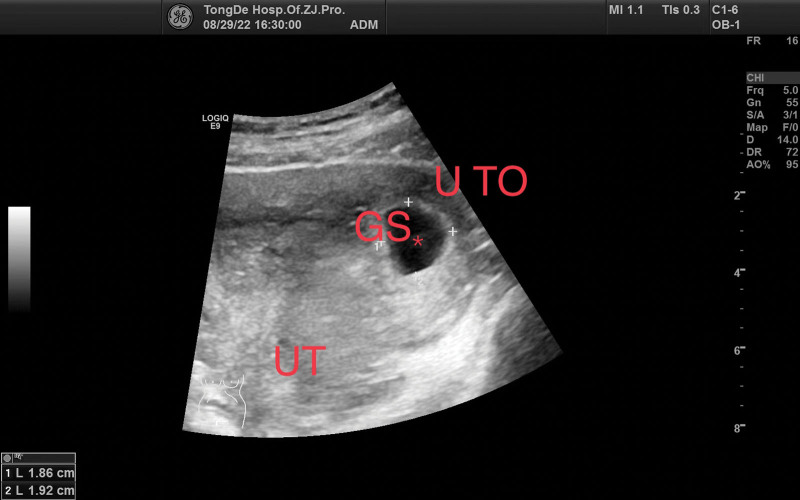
Gestational ultrasound before treatment (6 weeks, 3 days).
